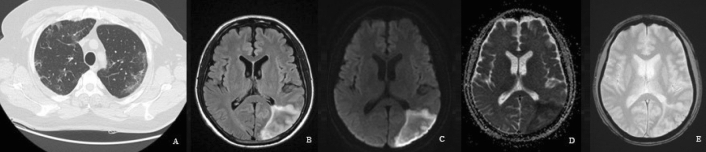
Fifty-eight-year-old male with RT-PCR proved SARS-CoV-2 pneumonia, (A) as revealed on chest CT. The patient also had right-sided weakness, right hemihyposthesia, and aphasia (stroke). Brain MRI was performed 4 days after the onset of the focal neurological manifestations as brain CT scanning was performed at the onset of such manifestations. (B) Axial FLAIR image showed increased signal intensity in the left parieto-occipital lobe (C) DW-MRI and (D) corresponding apparent diffusion coefficient (ADC) map showed diffusion restriction of the early sub-acute left parieto-occipital infarction. (E) No blooming was seen on two-dimensional (2D) T_2_* gradient-echo sequences (GRE), signifying no hemorrhage.
